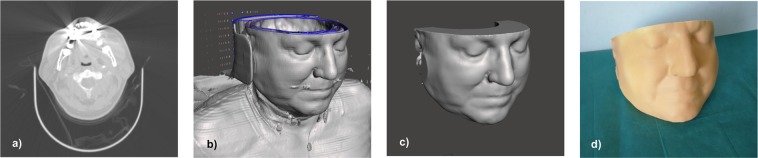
Examples taken from different data processing steps. Figure (a) shows an axial CT slice from the lower jawbone region taken from the one of the CT data sets. In (b), the skin surface, as reconstructed by the InVesalius software^19^, is shown. Figure (c) shows the generated 3D model, fitted for 3D printing, after manual post-processing. Finally, an exemplary 3D print is shown in figure (d). Informed consent for publishing these identifiable data was obtained from the participant.
